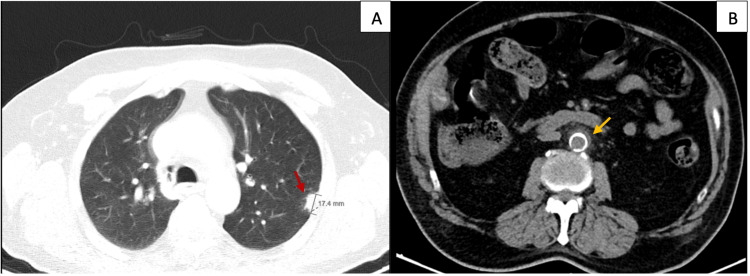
Computed tomography (CT) scan of Patient 2. (A) Chest CT scan revealing one of the newly identified pleural nodules on the left inferior lobe (red arrow). (B) Abdominal CT scan with periaortitis (densification of the fat adjacent to the infrarenal aorta, yellow arrow).
